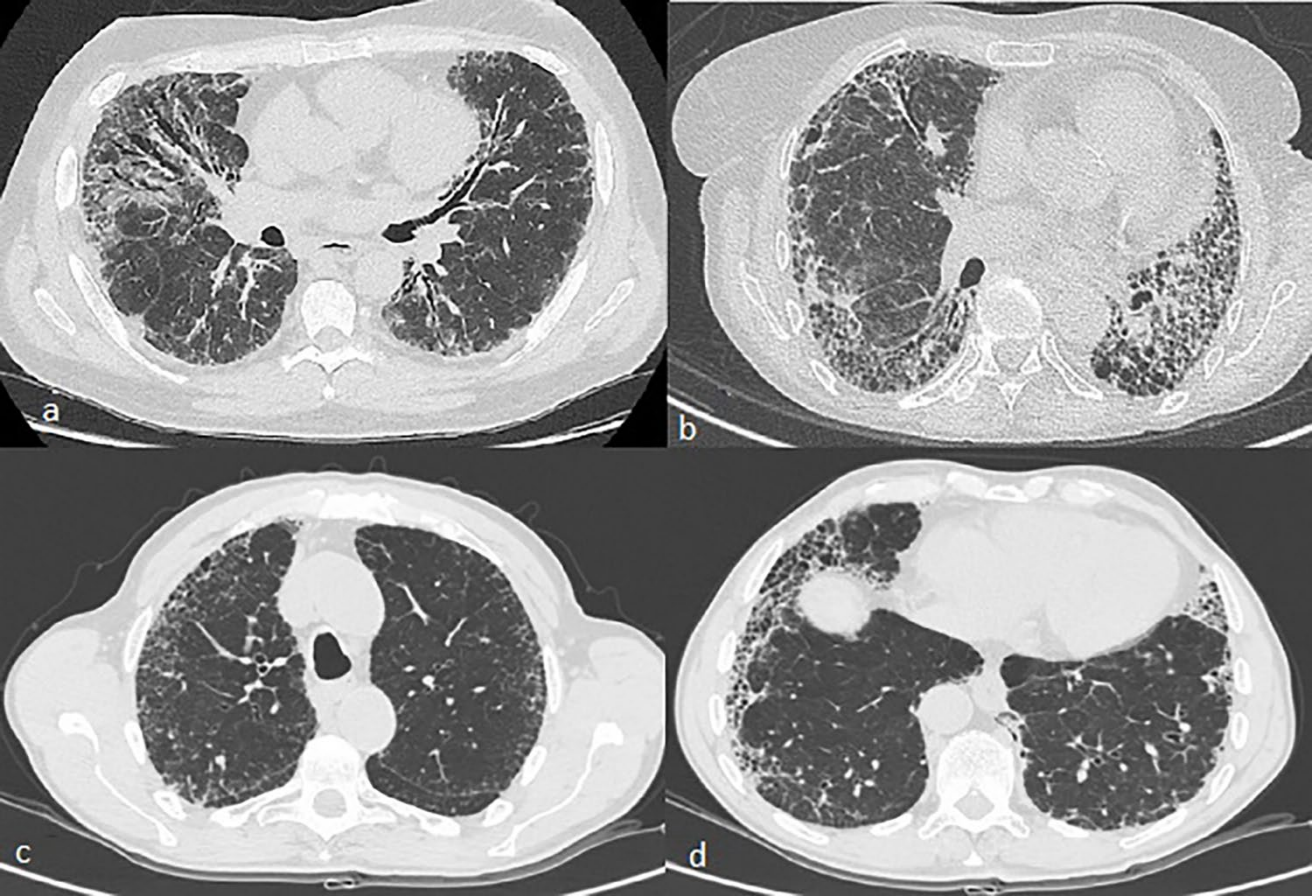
HRCT images. a: Fibrosing NSIP pattern in a patient with SSc. b: UIP pattern in a patient with overlap SSc-RA; c and d: UIP pattern in a patient with RA: HRCT shows reticular opacities and subpleural honeycombing
